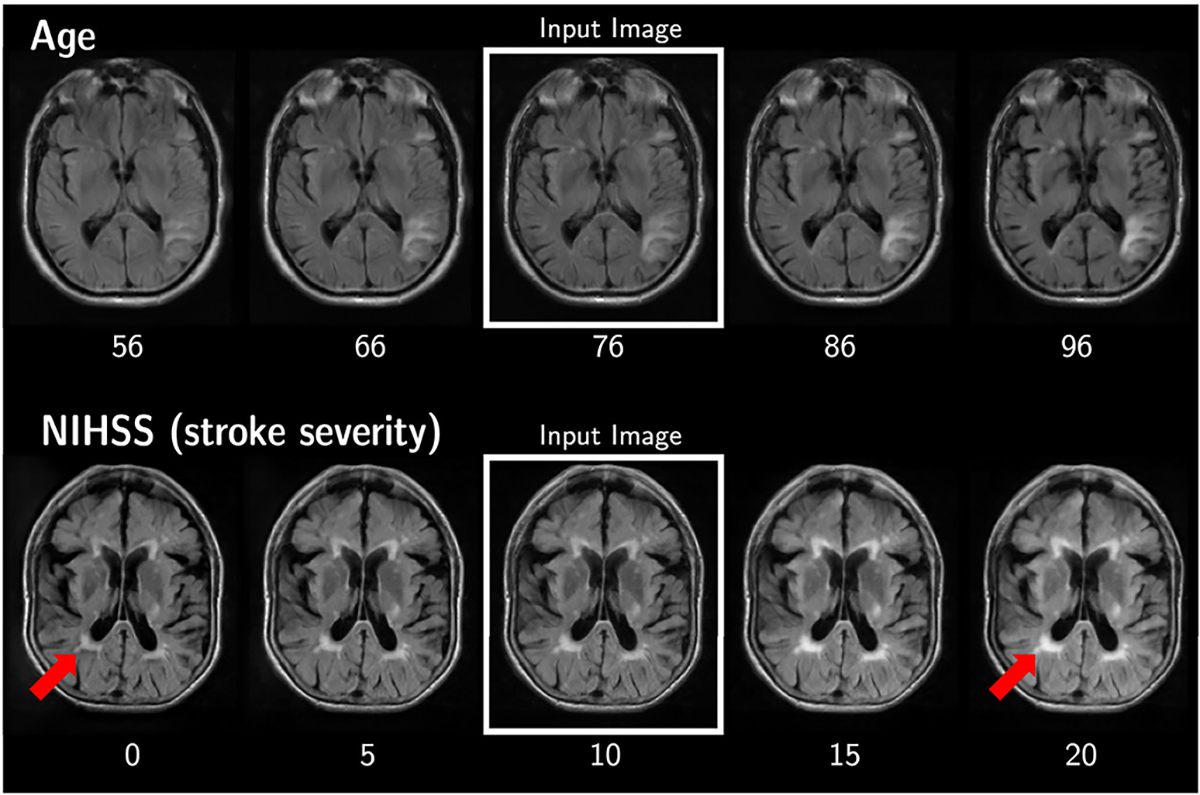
Synthetic MRIs generated by SIT-StarGAN, conditioned on two subjects’ scans in MRI-GENIE *(middle column)*. The generator transforms them into their neighboring images by conditioning on changes in age *(top row)* and stroke severity *(bottom row)*.
